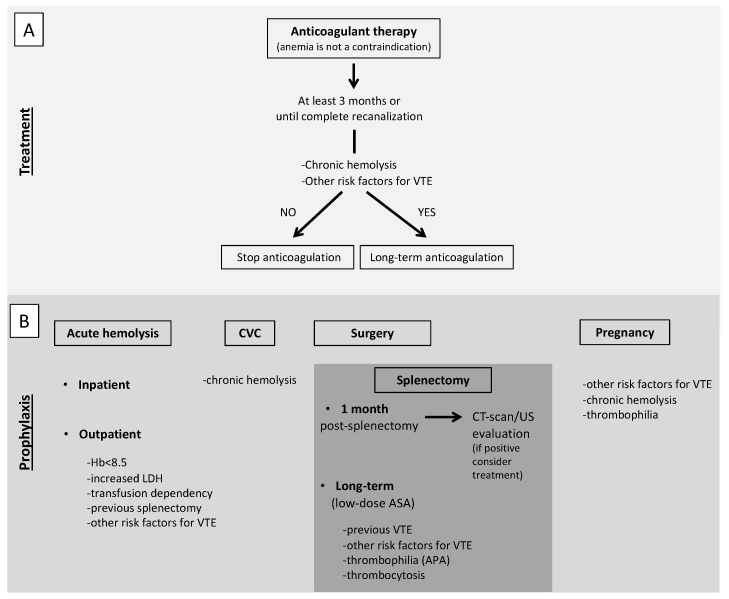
Proposed recommendations for treatment (A) and prophylaxis (B) of VTE in AIHA patients. (A). Anticoagulant therapy should be promptly started, regardless the degree of anemia, and continued for at least 3 months or until complete recanalization. Even if complete recanalization is reached, long-term anticoagulation should be considered for secondary prophylaxis of VTE, evaluating the presence of persistent risk factors for VTE. (B). Antithrombotic prophylaxis with low-molecular-weight heparin is recommended for inpatients with acute hemolysis and should be considered for outpatients with acute hemolysis associated with severe anemia, particularly in splenectomized patients or in the presence of concomitant risk factors for VTE. In patients with acute hemolysis undergoing central venous catheter positioning, antithrombotic prophylaxis should be started. We suggest antithrombotic prophylaxis for all patients with chronic immune-mediated hemolysis. In patients undergoing splenectomy, post-operative antithrombotic prophylaxis with low-molecular-weight heparin should be started, unless contraindicated and continued for at least one month when an objective evaluation should be performed before its withdrawal. Long-term anticoagulation or low-dose aspirin should be taken into account in patients with previous VTE or concomitant risk factors for VTE. In pregnant women with chronic hemolysis, antithrombotic prophylaxis should be started, especially in the presence of other risk factors of VTE or thrombophilia abnormalities. VTE, venous thromboembolism; AIHA autoimmune hemolytic anemia; CVC, central venous catheter; CT-scan, computed tomography scan; US, ultrasound; ASA, acetylsalicylic acid; APA, antiphospholipid antibodies.
